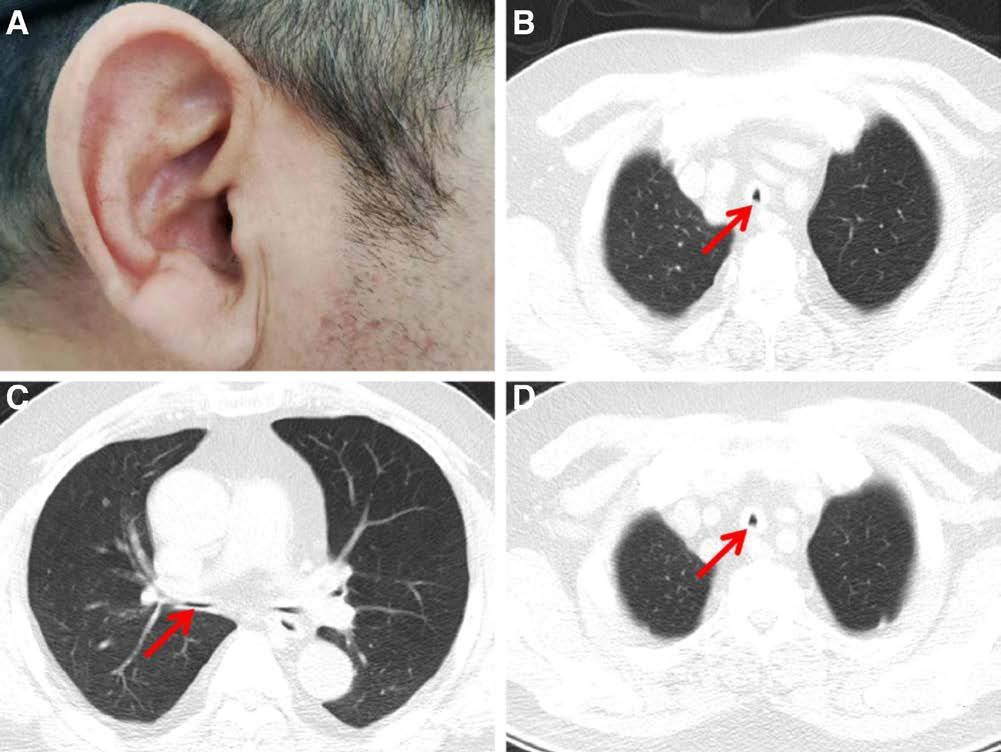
(A) Onset of cartilage collapse and swelling in the patient’s ears. (B) CT plain scan shows diffuse thickening of the tracheal and bronchial walls, with the red arrow indicating the airway wall thickening. (C) CT plain scan identifies airway stenosis and collapse, with the red arrow signifying the main airway collapse. (D) The red arrow points the bronchial collapse. CT = computed tomography.
